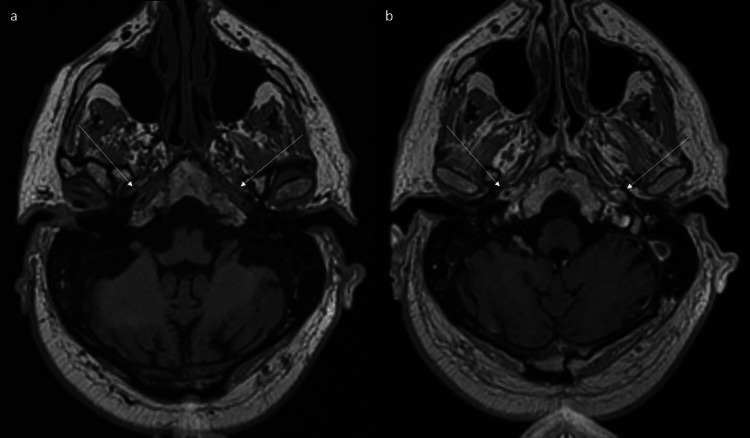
MRI shows abnormal wall thickening and enhancement of petrous portion of internal carotid arteries. (a) Axial non-contrast and (b) contrast-enhanced T1 space sequences show abnormal wall thickening and enhancement of petrous portion of internal carotid arteries (arrows).
